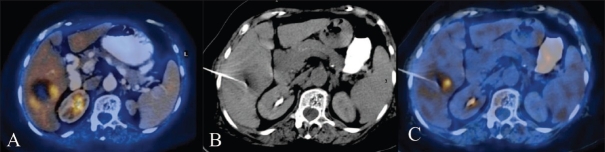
Axial fused PET/CT image in a 57-year-old woman demonstrates a necrotic mass in the right lobe of the liver and a small focus of increased metabolic activity along the wall, which is more along the medial aspect. CT scan (B) shows the needle tip in place. The accuracy of positioning of the needle tip in the most metabolically active medial portion is confirmed on the axial fused PET/CT image. The diagnosis was hepatoma
